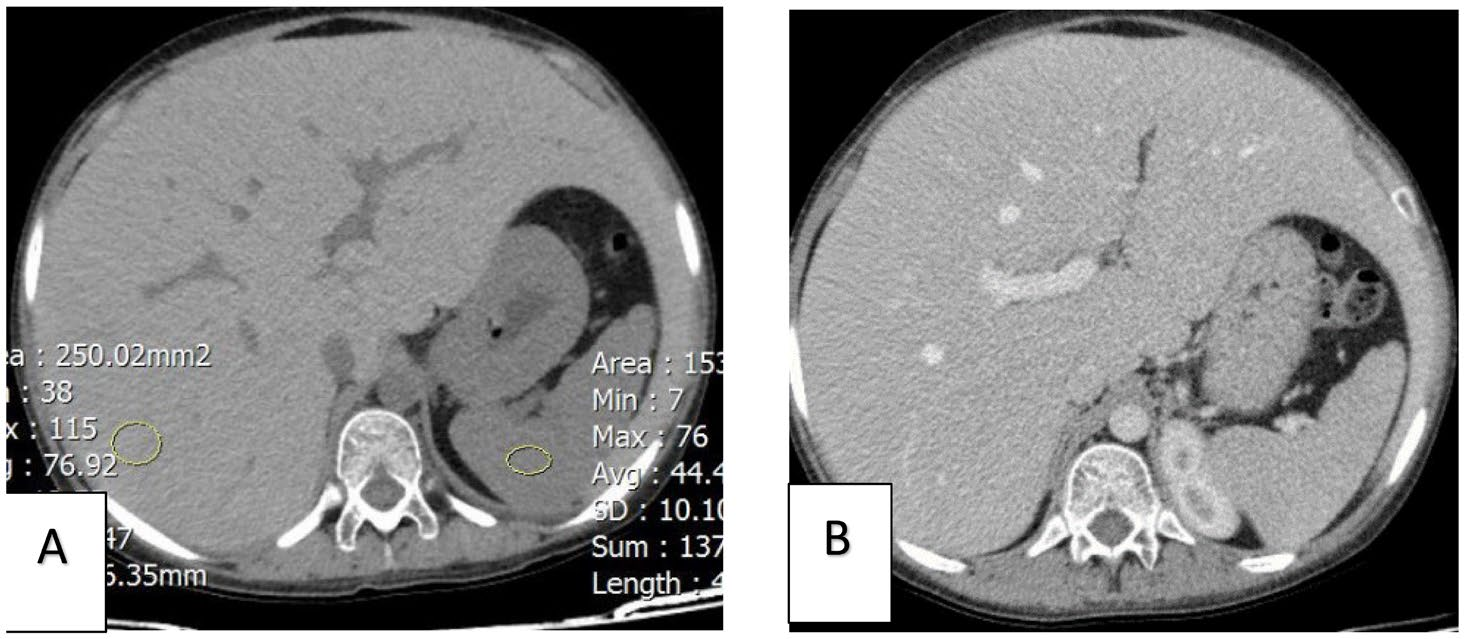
(A) Computed tomography (CT) scan of abdomen without contrast and (B) with contrast depicting hepatomegaly and diffuse increase in liver density (in non‐contrast CT scan) with no focal lesion.
